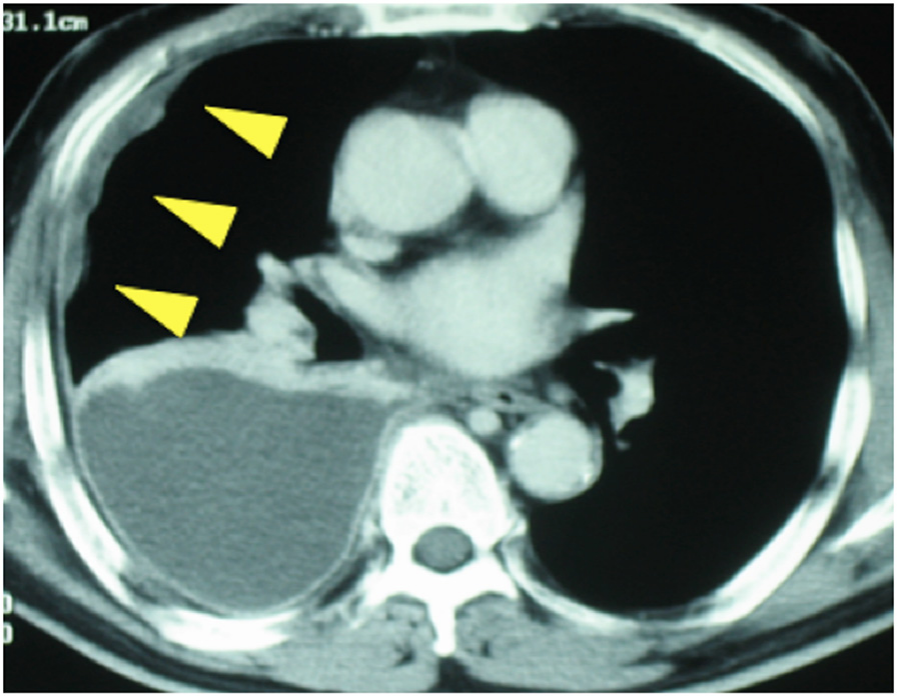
Computed tomography images before treatment. A chest computed tomography scan before treatment shows right pleural diffuse thickening (arrows).
